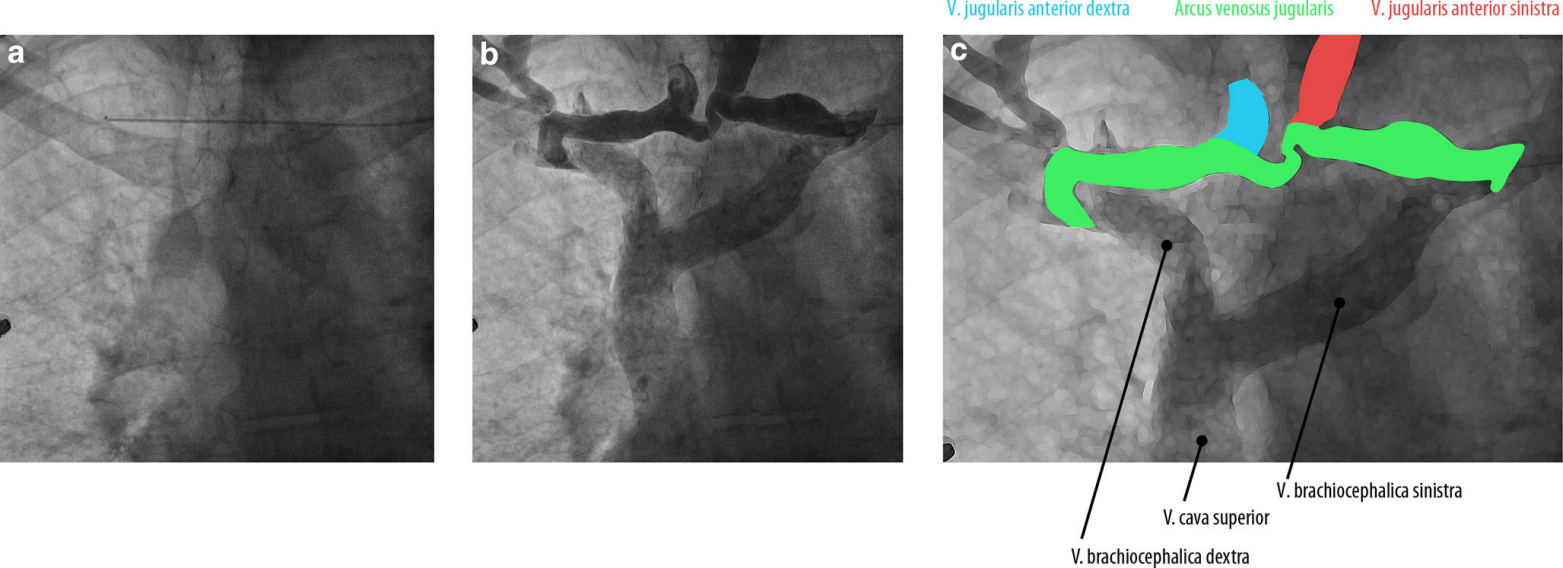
a Chest radiography showing a guide wire (arrow head) running horizontally from the left subclavian vein to the contralateral side. b An overlay of sequential chest X‑rays showing a phlebogram of two anterior jugular veins running parallel *(venae jugulares anteriores)* which are connected caudally by a jugular arch *(arcus venosus juguli)*. See also the online video
